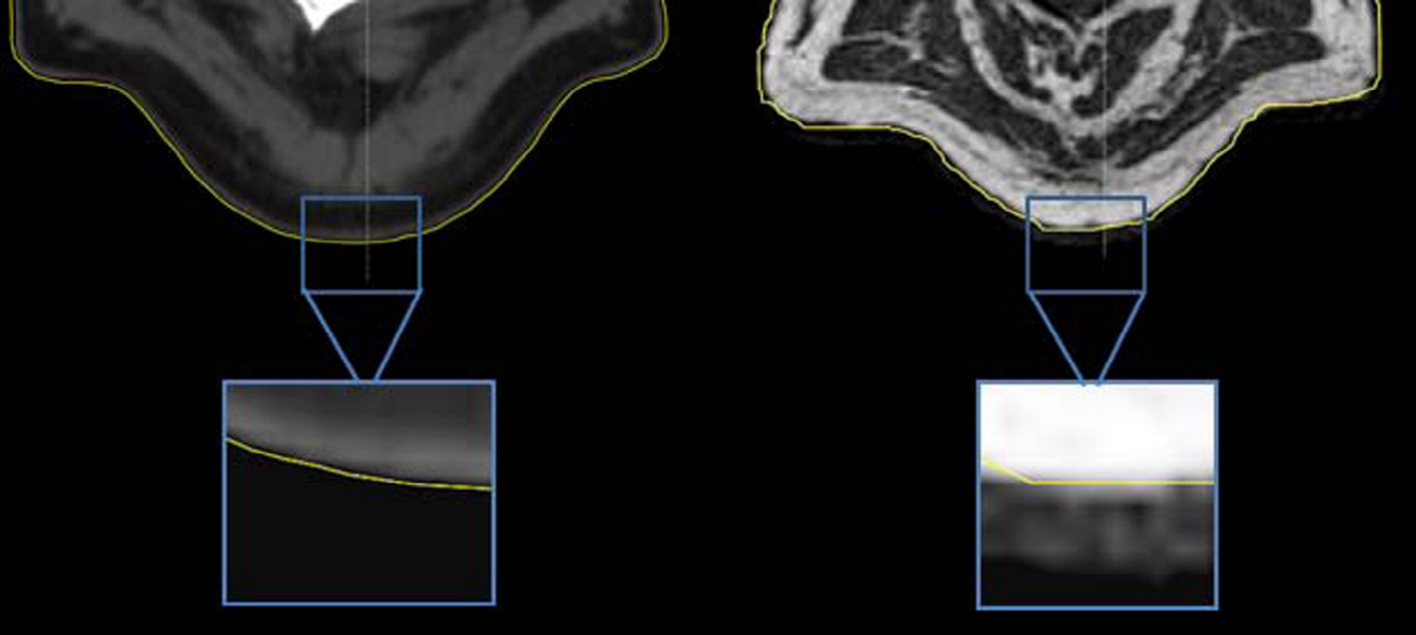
Zoom on CT (left) and on MR (right) images on the patient's boundary. The external contour is represented in yellow. (For interpretation of the references to colour in this figure legend, the reader is referred to the web version of this article.)
